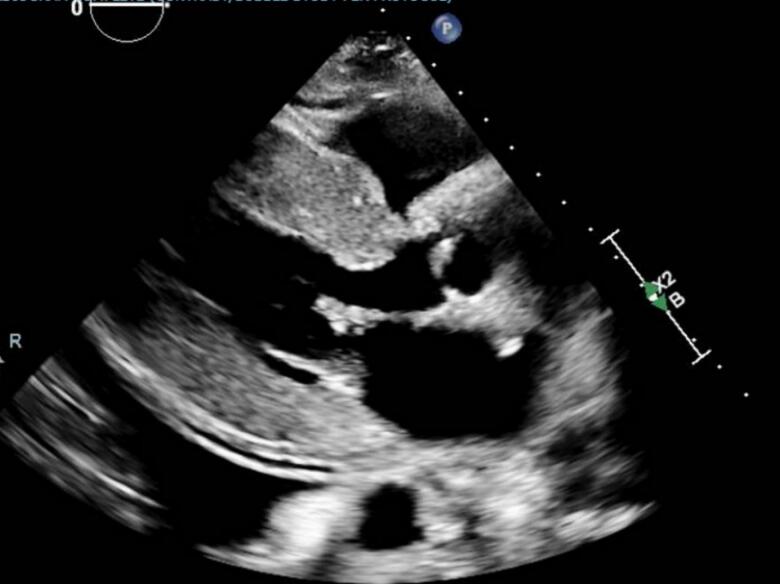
Echocardiography demonstrating left ventricular hypertrophy.
